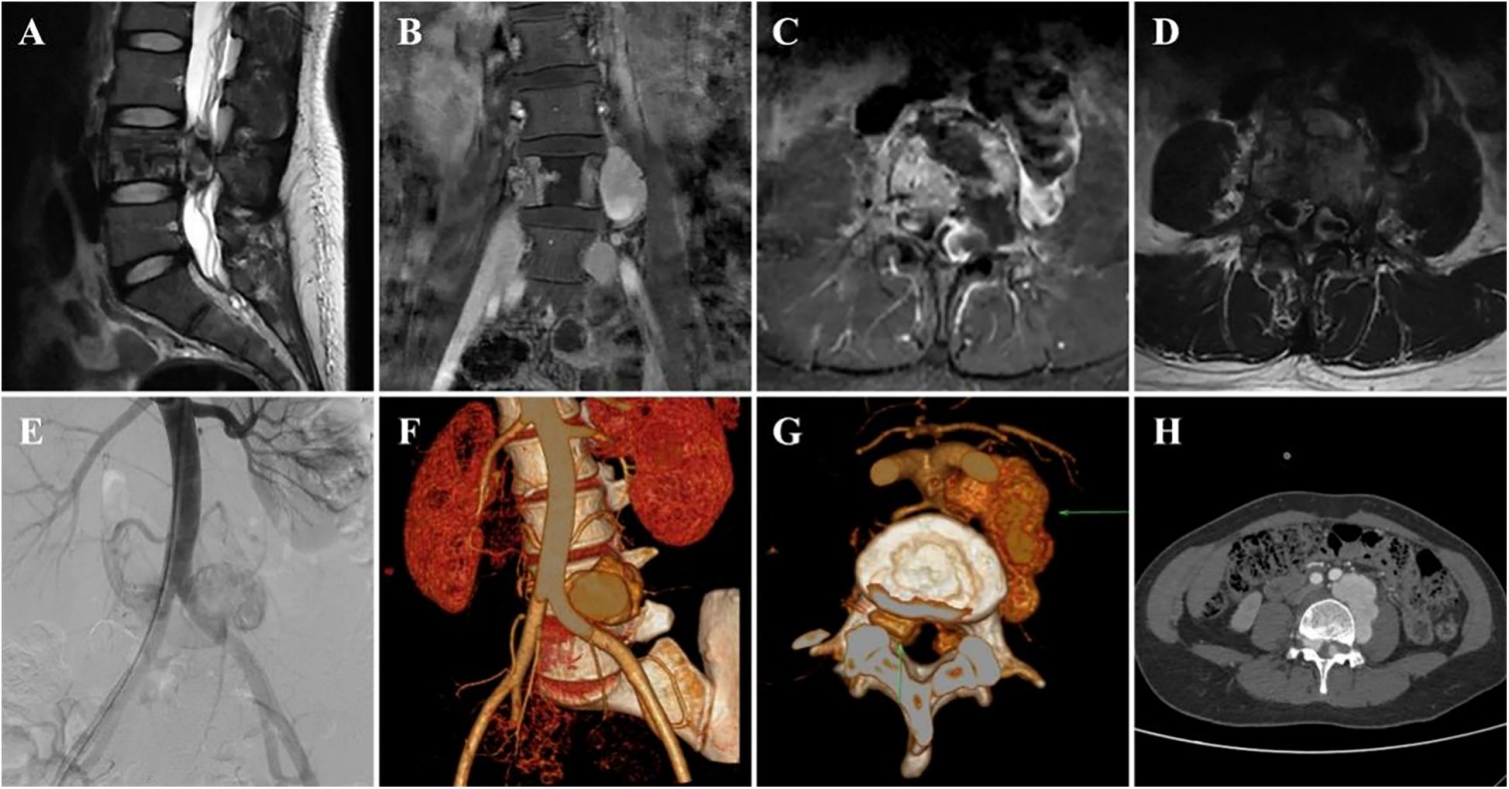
Preoperative imaging data of the patient. (A–D) Preoperative MRI images (E), preoperative DSA image, and (F–H) preoperative CTA image.
